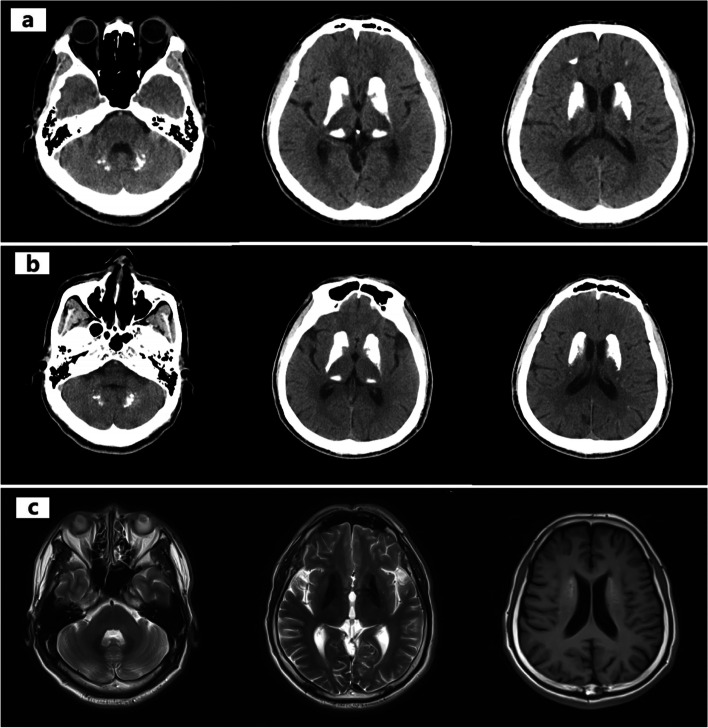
CT and MRI of the proband (II: 3). a and b were taken on 2020/08/04 and 2021/05/28, respectively. Both CT scans showed multiple calcifications in the head of the bilateral caudate nucleus, bilateral thalamus, bilateral cerebellum, and bilateral parietal lobes, and there was no significant difference between the two lesions. c shows the MRI. Symmetrical abnormal signals in the bilateral dorsal thalamus, basal ganglia and dentate nucleus of the cerebellar hemisphere are considered to be paramagnetic deposits
